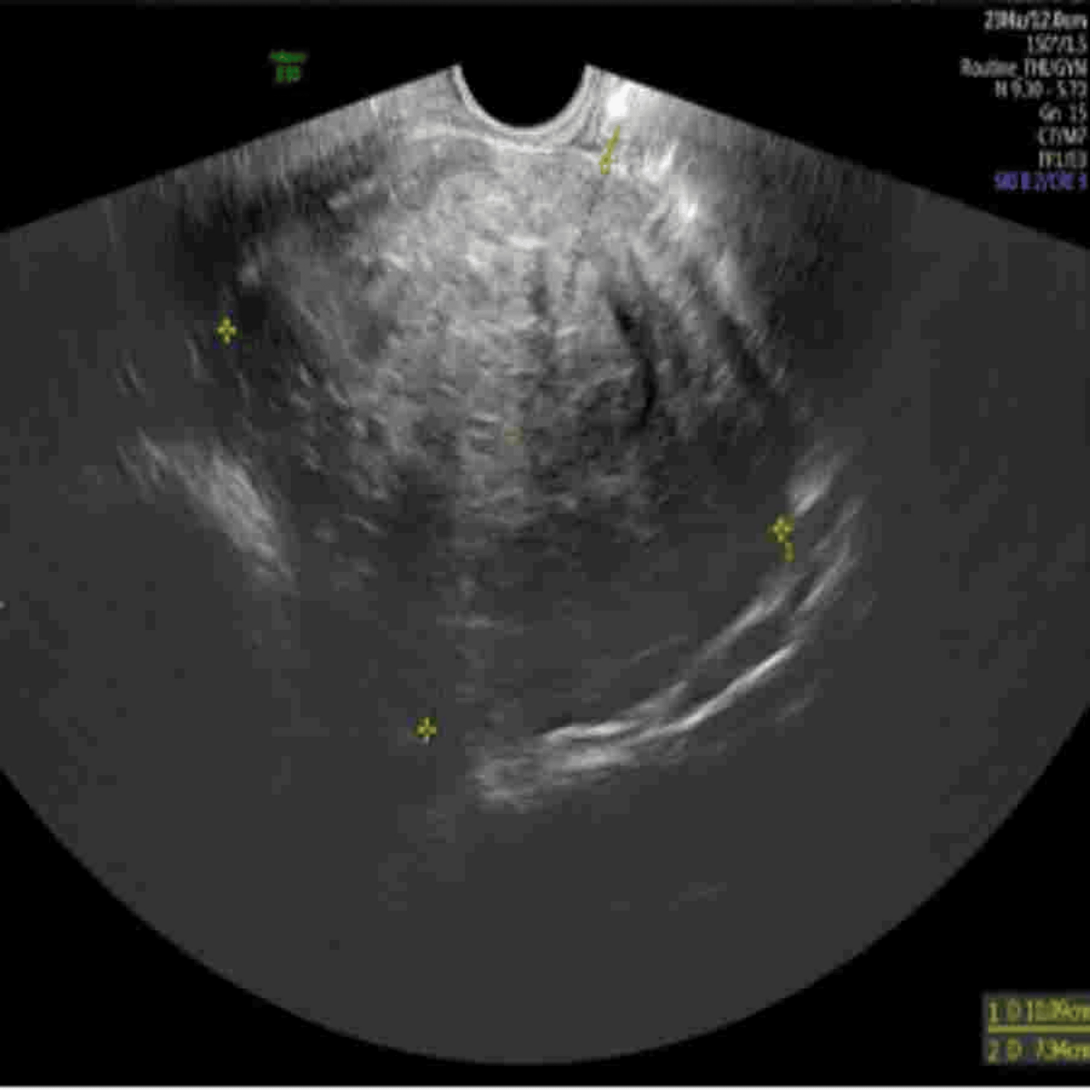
Transvaginal ultrasound showing a large hypoechoic cervical fibroid measuring approximately 10.09×7.94 cm, distorting the lower uterine segment and obscuring visualization of the internal cervical os.
